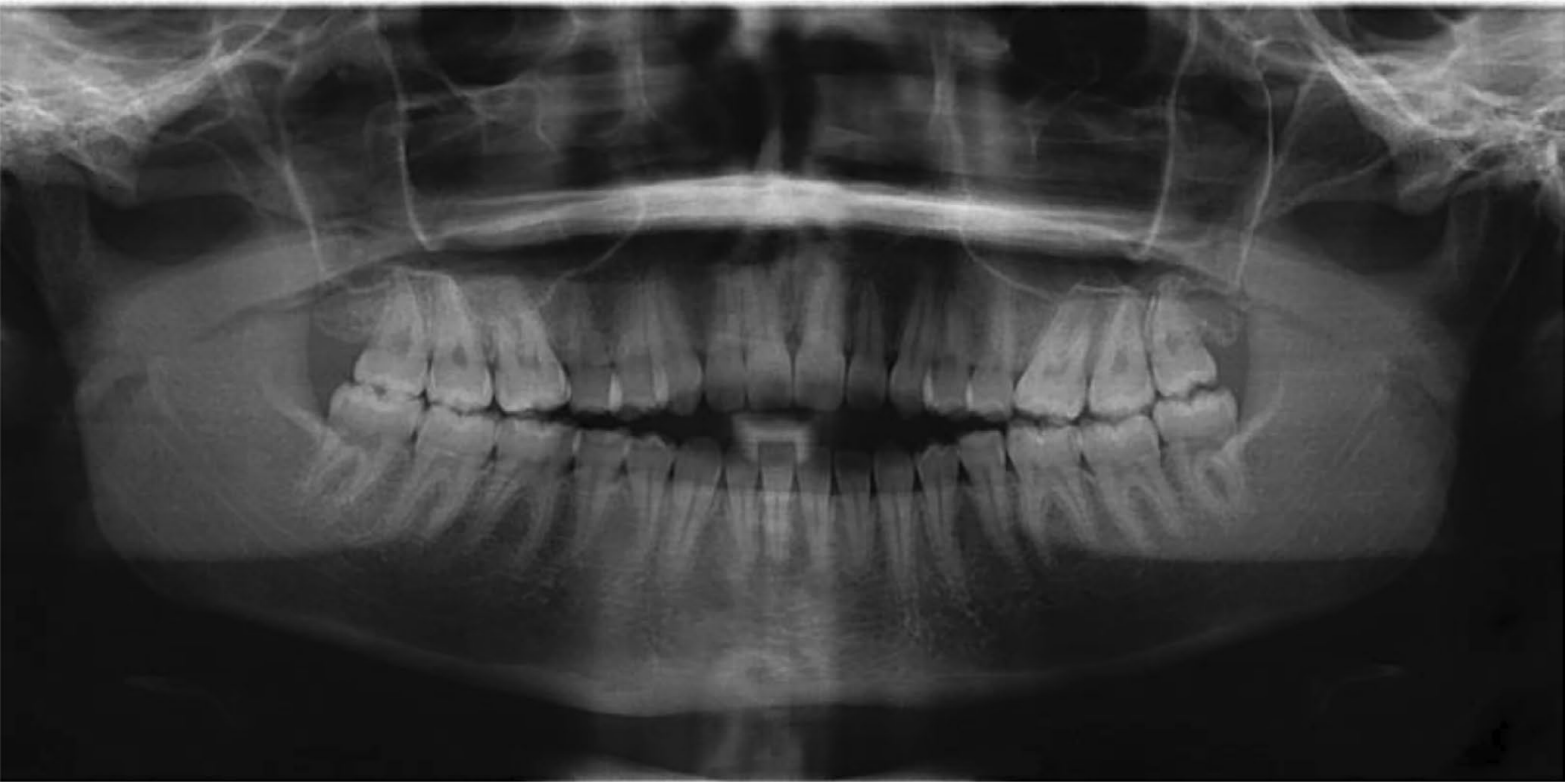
Pre‐treatment panoramic radiograph shows third molars are present.
